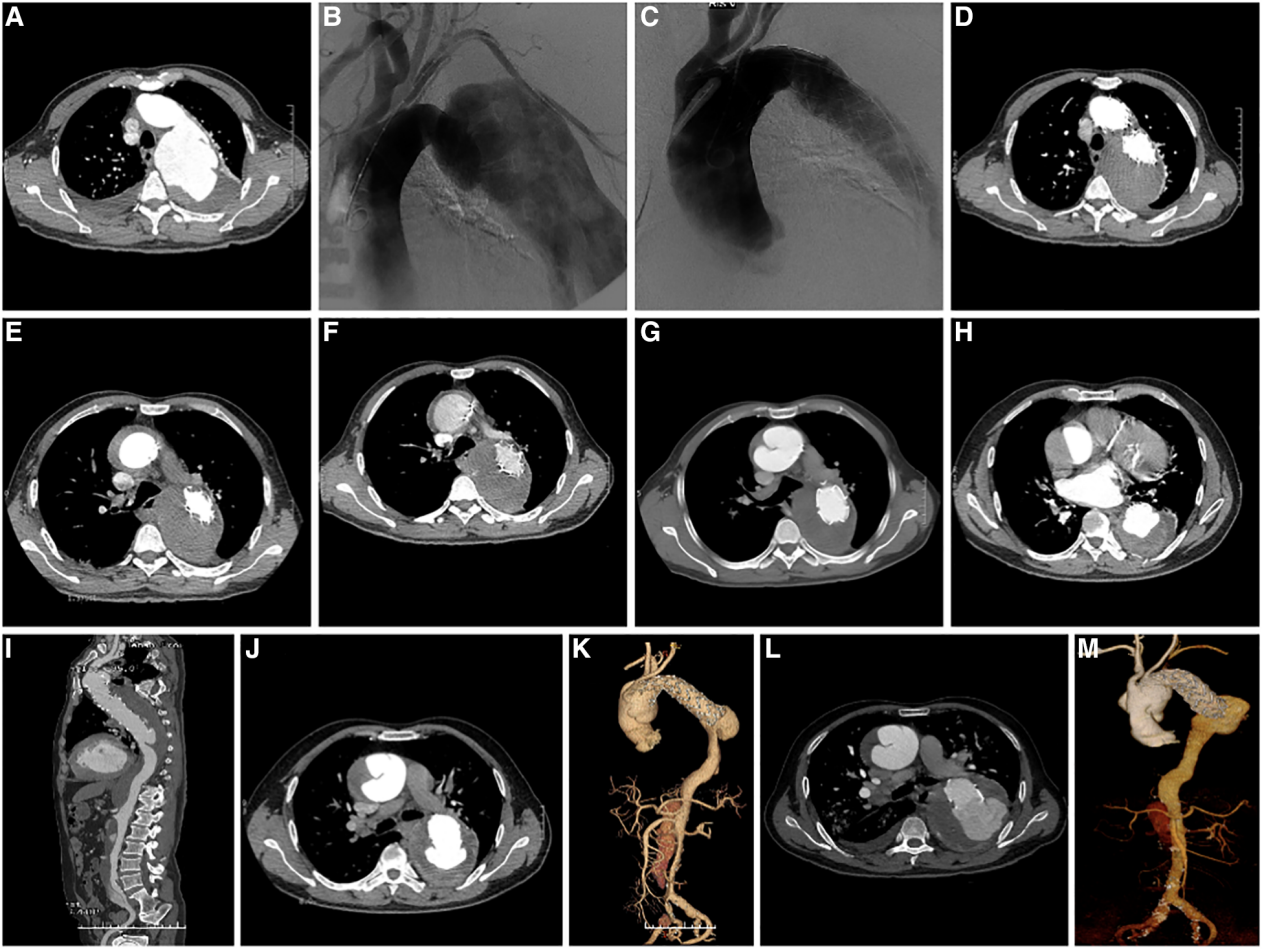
(A) preoperative CTA axial image of TEVAR showed TBAD; (B) Pre-stenting DSA shows a large false lumen with significant compression of the true cavity, and the entry located distal to the LSA; (C) DSA after stenting showed complete occlusion of the entry, widening of the true lumen, and improved blood flow; (D) CTA axial image ten days following TEVAR showed complete occlusion of the entry, thrombosis of the false lumen, and good visualization of the true lumen; (E) CTA at 13 days after TEVAR showed intermural hematoma formation in the ascending aorta; (F) CTA at three weeks after TEVAR showed intermural hematoma formation in the ascending aorta, with no significant change compared to the previous CTA; (G) CTA at 20 weeks after TEVAR showed progression of ascending aortic coarctation; (H) axial image of CTA at 32 months after TEVAR showed proximal RTAD and distal SINE; (I) 3D reconstruction of CTA at 32 months after TEVAR showed proximal RTAD and distal SINE; (J) axial image of CTA at 46 months after TEVAR showed progression of proximal RTAD and distal SINE. (K) 3D reconstruction of CTA at 46 months after TEVAR showed progression of both proximal RTAD and distal SINE; (L) Axial images of CTA at 56 months after TEVAR showed progression of both proximal RTAD and distal SINE; (M) 3D reconstruction of CTA at 56 months after TEVAR showed progression of both proximal RTAD and distal SINE. CTA, CT angiography; TEVAR, Thoracic Endovascular Aortic Repair; RTAD, Retrograde Type A Aortic Dissection; 3D, three dimension; SINE¸Stent Induced New Entry.
